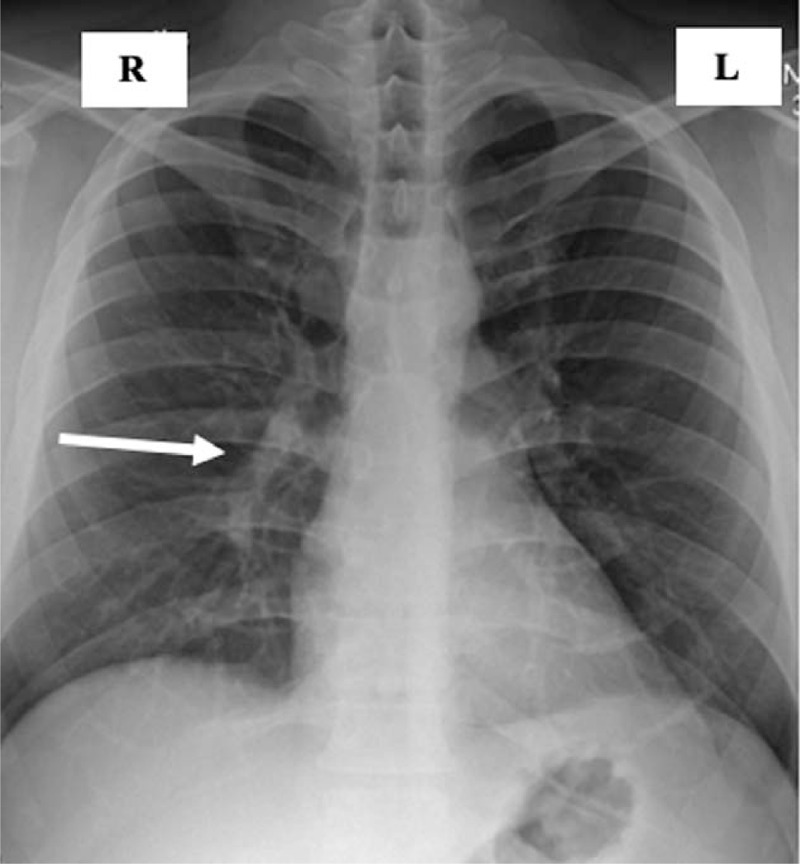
Chest X-ray on hospital admission. Inflammatory changes are observed in the middle and lower lung fields on the right (white arrow).
